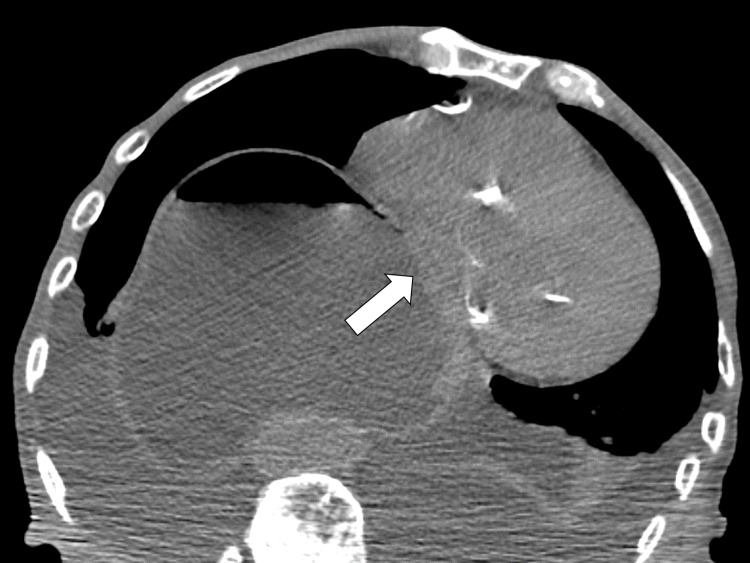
Abdominal CT scan on the fifth day The CT revealed a giant esophageal hiatal hernia compressing the heart anteriorly. CT, computed tomography
